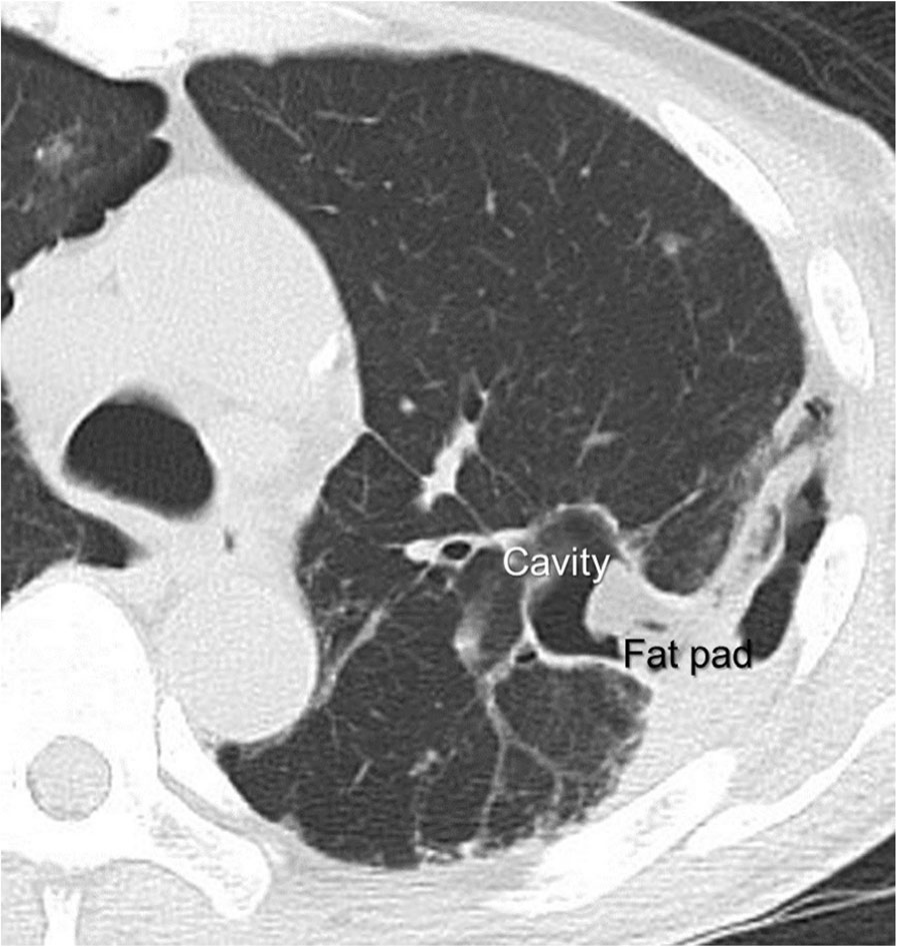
Postoperative chest CT demonstrated that the pulmonary cavity was occluded by the PFP
